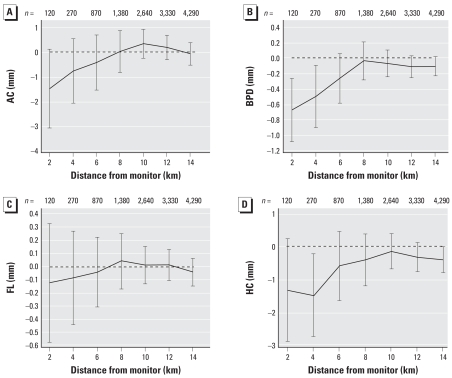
Change in ultrasound measurements (mm) for an 0.8-ppb increase in SO_2_ during days 0–30 of gestation by distance to monitor. (*A*) AC; (*B*) BPD; (*C*) FL; (*D*) HC.
